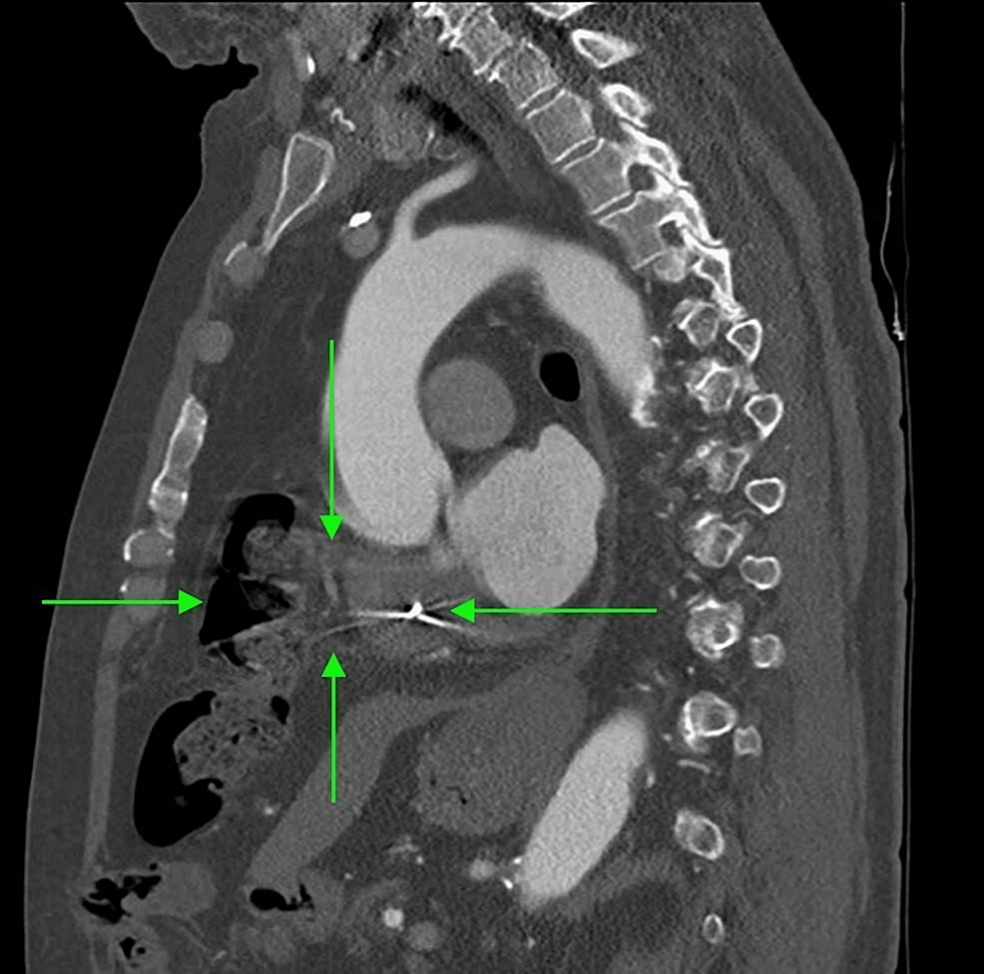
CTA of the chest revealing diaphragmatic herniation CTA: computed tomography angiogram.
